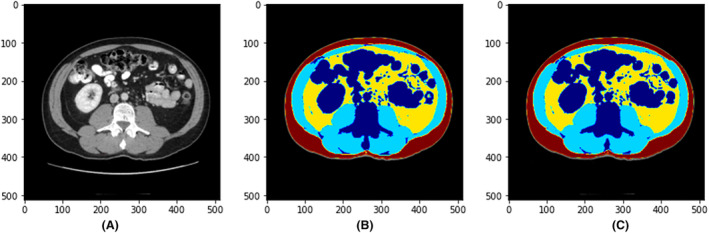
A sample case demonstrating the original CT slice (A), the manual segmented single slice (B) and the AI‐segmented single slice (C).
